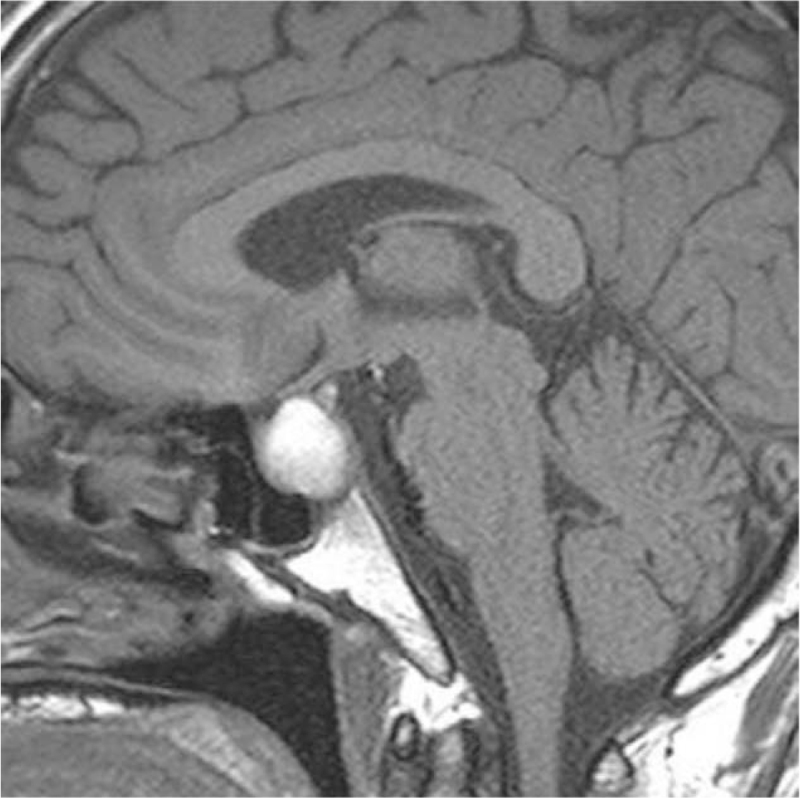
Sagittal view of T1-weighted sellar MRI: a pituitary macroadenoma with mild displacement of the optic chiasm. MRI = magnetic resonance imaging.
